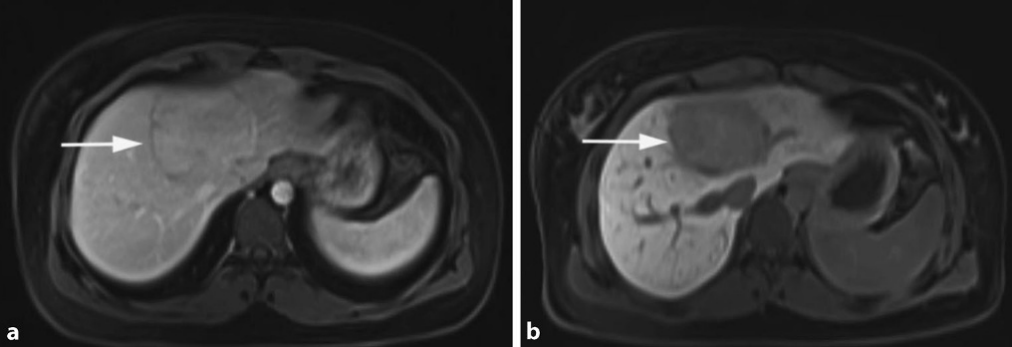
HCA: axial venous phase MRI with Primovist® (Bayer Schering Pharma, Berlin, Germany) shows an only slightly hyperintense lesion (*arrow*) in the centre of the liver (a) with a marked capsule. In the hepatobiliary phase no uptake of contrast agent is seen within the lesion (*arrow*), which is further consistent with HCA (b) [9]
